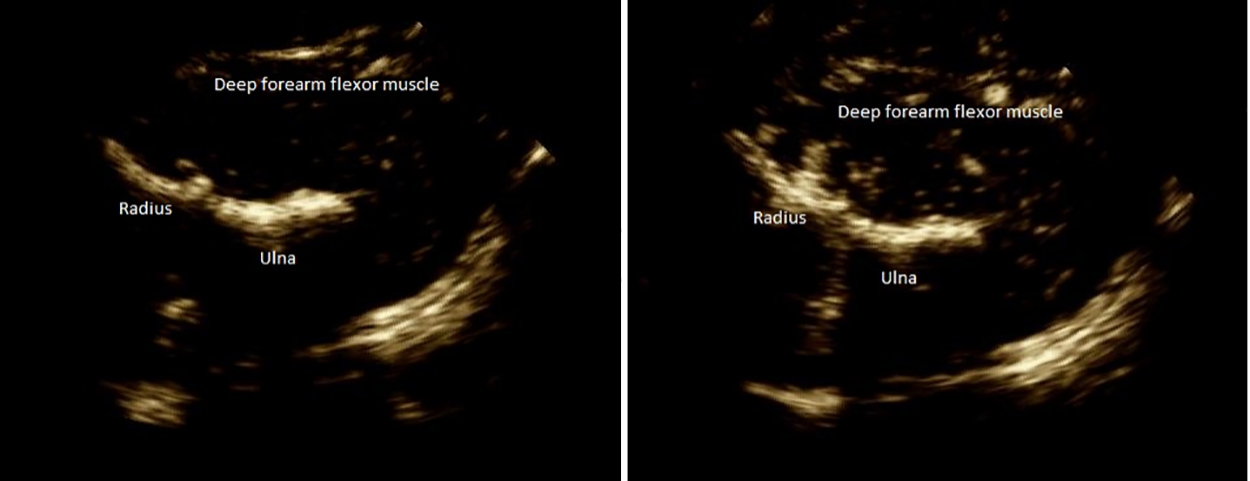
Deep forearm flexor muscle at baseline (Panel A) and after contrast media infusion (Panel B). Small white points detectable in Panel B are generated by reflection of microbubbles when insonated by ultrasound beam.
